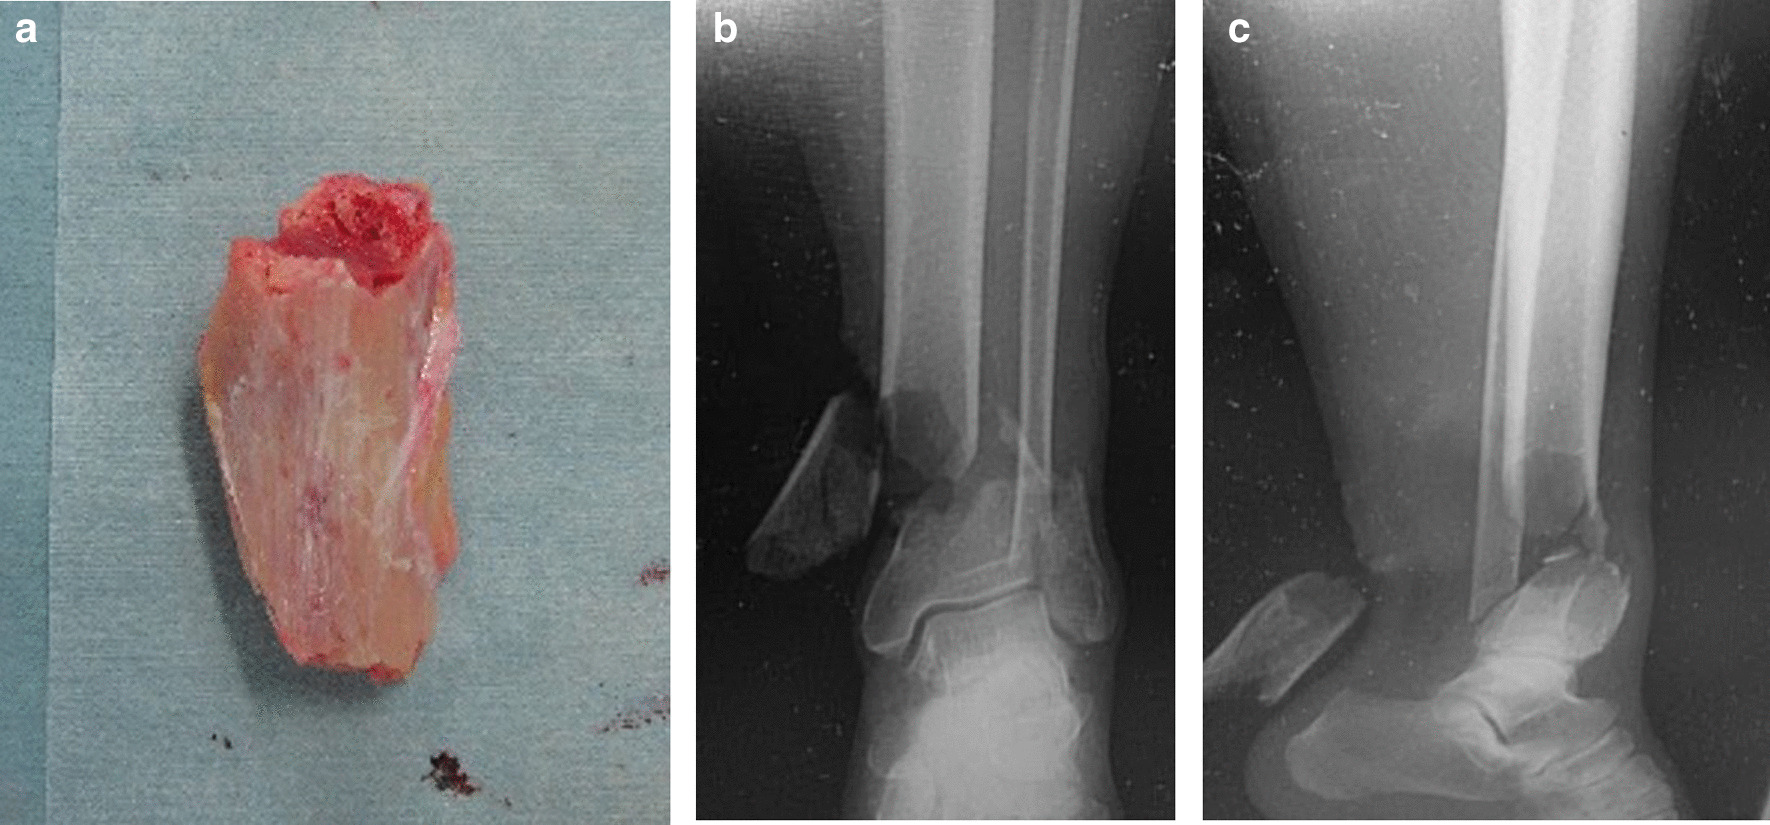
a The contaminated large bone that fell out of the body; b and c X-ray images taken in the emergency room
